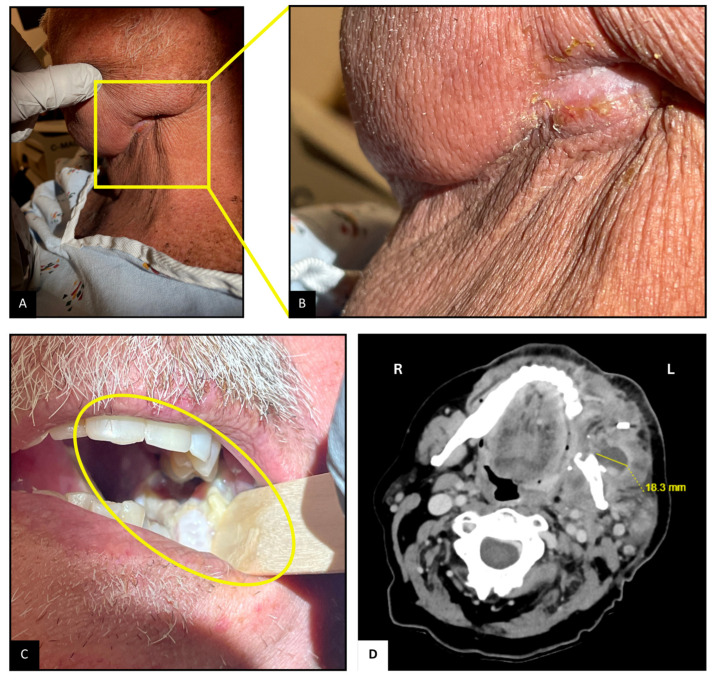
Neck and Oropharyngeal Examination and Imaging. (A,B): Scabbed incision below the left mandibular angle. (C): Sloughing and mucositis at the left retromolar trigone; flap caught in occlusion line. (D): CT scan showing 2.3 × 1.8 cm^2^ complex abscesses at the left retromolar region, with associated mandibular irregularity.
